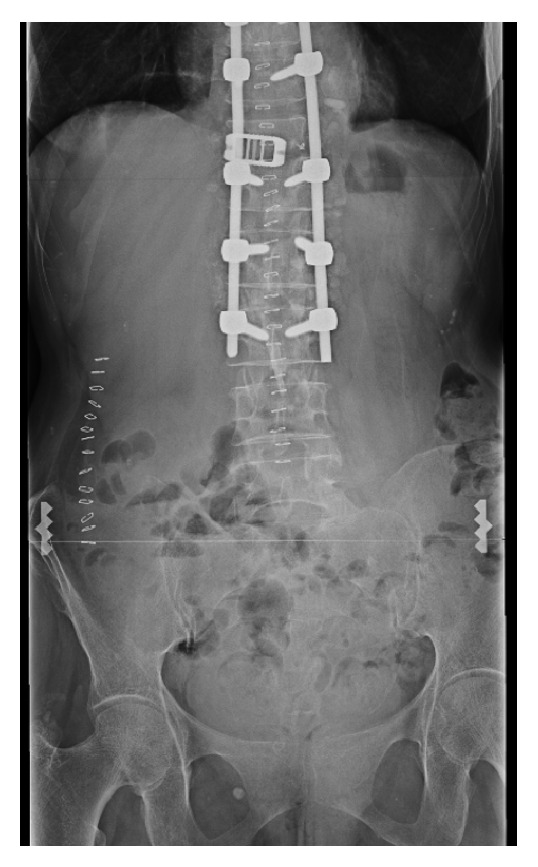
Initial iliac crest bone harvest procedure postoperative radiograph demonstrating relative position of incision with closure staples.
